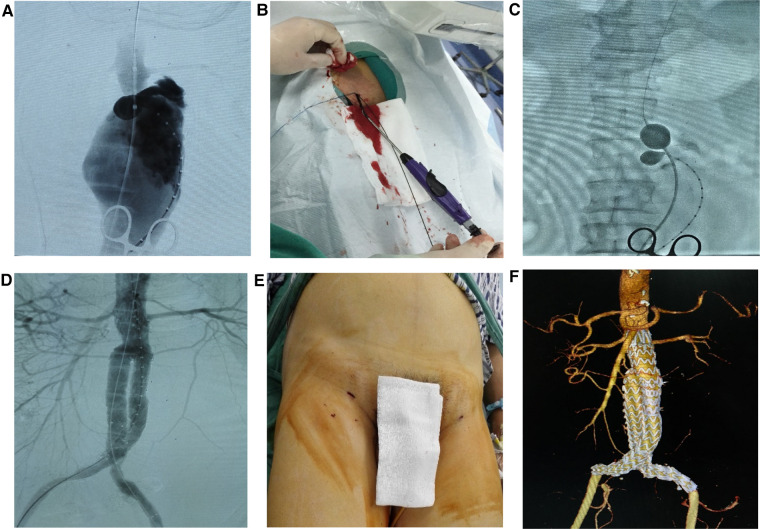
Ruptured percutaneous (rPEVAR) operative procedure (A) angiogram of ruptured AAA. (B) Percutaneous cannulation and deployment of ProGlide devices in the bilateral CFAs. (C) A stiff Lunderquist wire and a12Fr 45 cm sheath were advanced to the perivisceral aorta then a Coda balloon was advanced through the sheath and inflated in the distal thoracic aorta. (D) EVAR was performed. (E) The sheaths were removed and the femoral cannulation sites were closed using the pre-deployed ProGlide devices. (F) Follow-up 3D CTA 30-day post EVAR.
